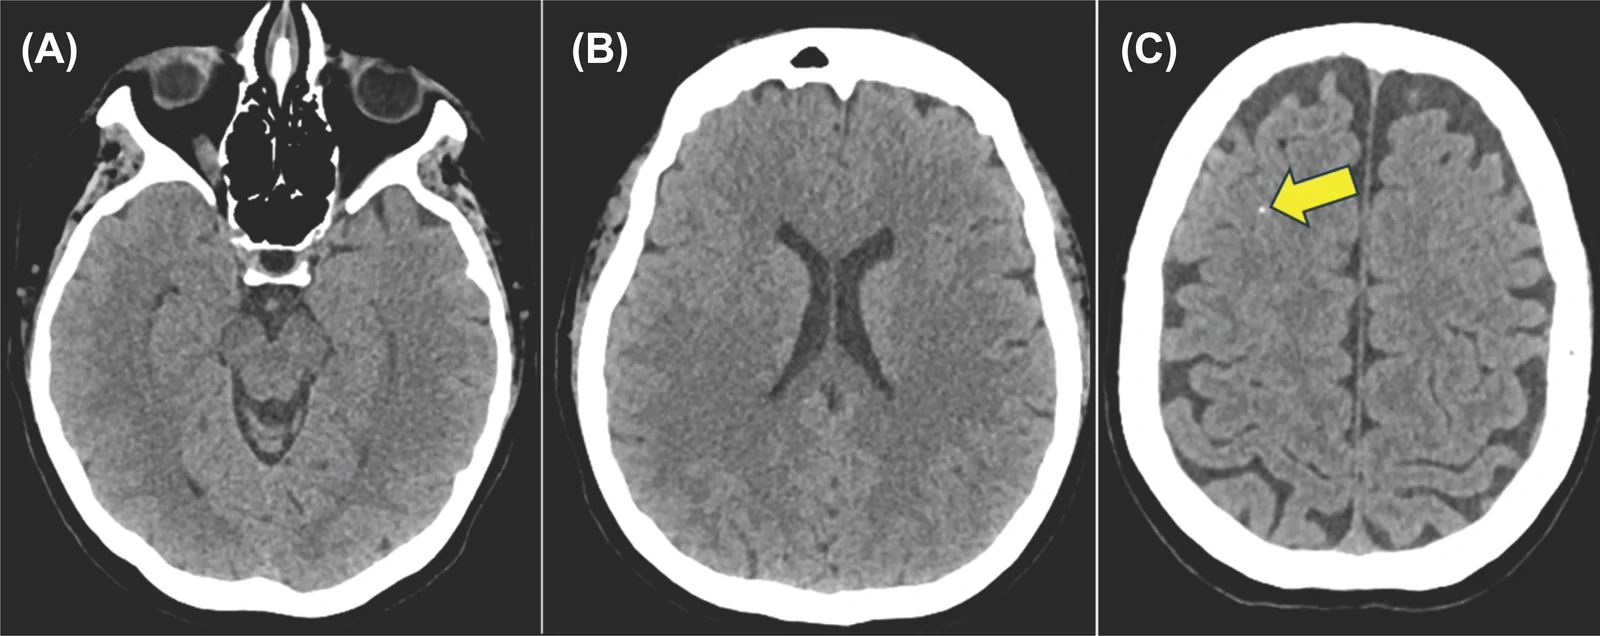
Non-contrast head CT. Multiple small cortico-subcortical calcifications in both hemispheres (yellow arrow: right hemisphere). No acute hemorrhage or mass effect. Abbreviation: CT, computed tomography.
